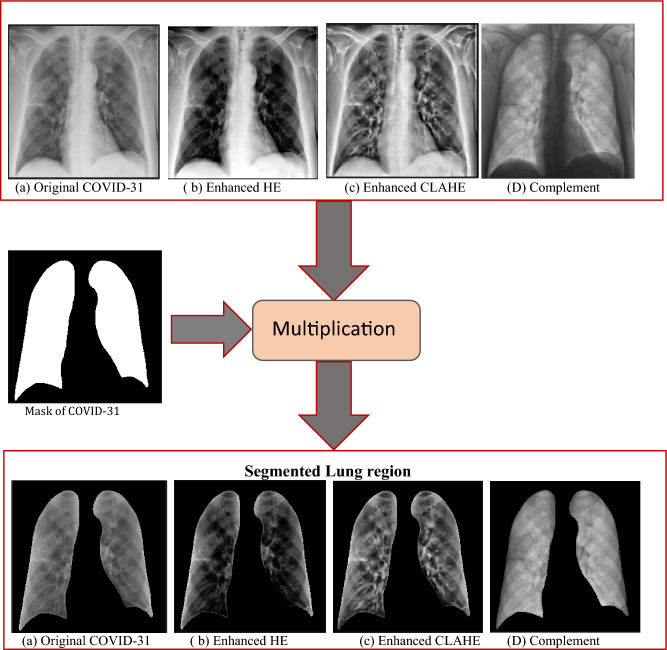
X-ray original image and its enhanced versions and the segmented lung region of each version.
